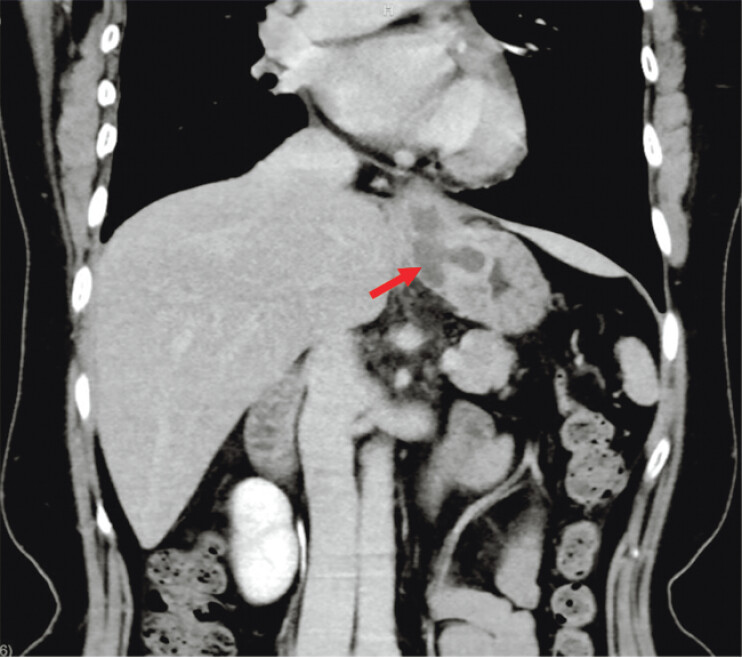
Contrast-enhanced computed tomography of the abdomen with coronal reconstruction
